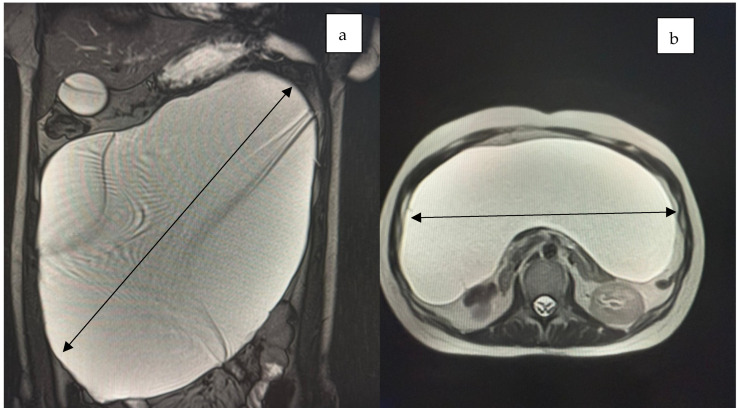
The axial ((a), black double arrow) and cross-section ((b), black double arrow) MRI scans of the abdomen revealed a tumor mass occupying the entire peritoneal cavity with fluid content.
